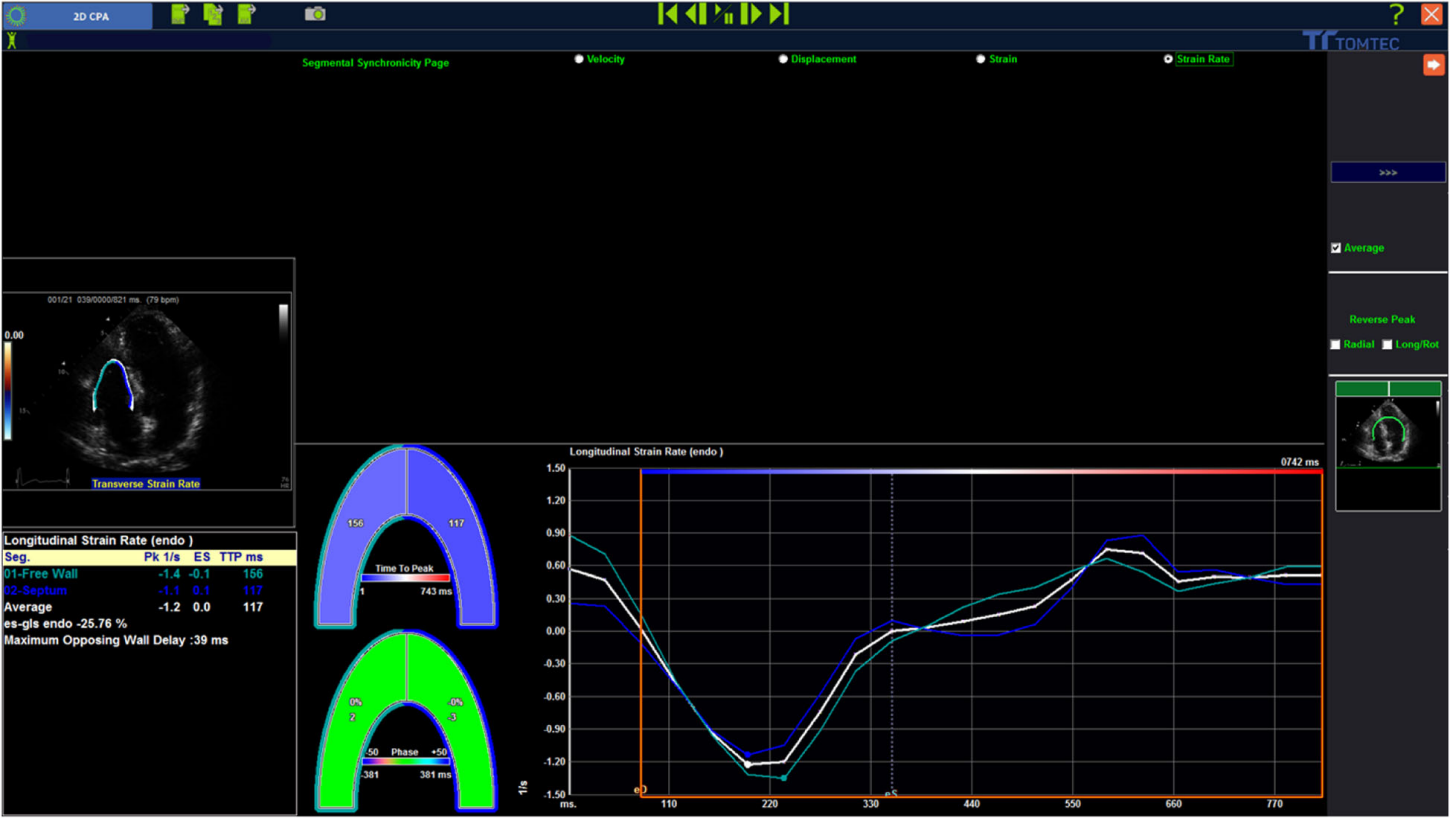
RV strain analysis using the TomTec software (2D cardiac performance analysis) for RVGLS and RVFWS by speckle tracking echocardiography. Mean frame rates = 70–90 frames/s. Digitally stored for 3 cardiac cycles. Endocardial border tracking was achieved automatically using 2 points in the annular region and 1 point in the apical segments. Tracking quality was visually verified. Segments that failed initial tracking were manually adjusted. Segments that could not be tracked properly after manual adjustment were rejected. RV GLS strain was measured from the average value of the longitudinal peak systolic strain of the RV free wall and the RV septal wall in the apical 4 chamber view. LVEF: Left ventricular ejection fraction. RVFAC: Right ventricular fractional area change. RVFWS: Right ventricular free wall strain. RVGLS: Right ventricular global longitudinal strain
